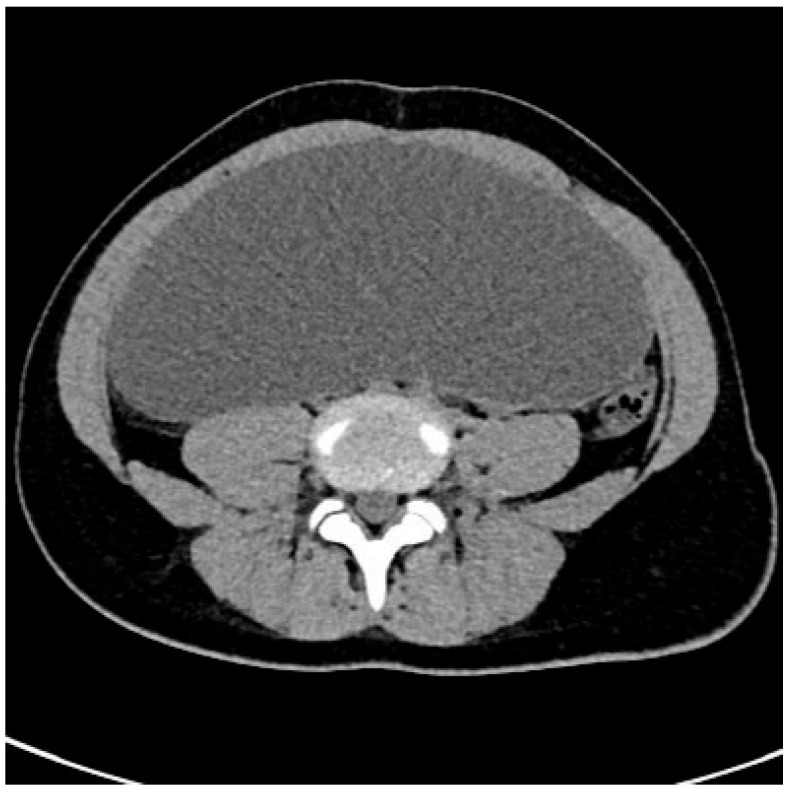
The abdominal CT scan revealed a large tumor in the pelvis and mid-abdomen.
